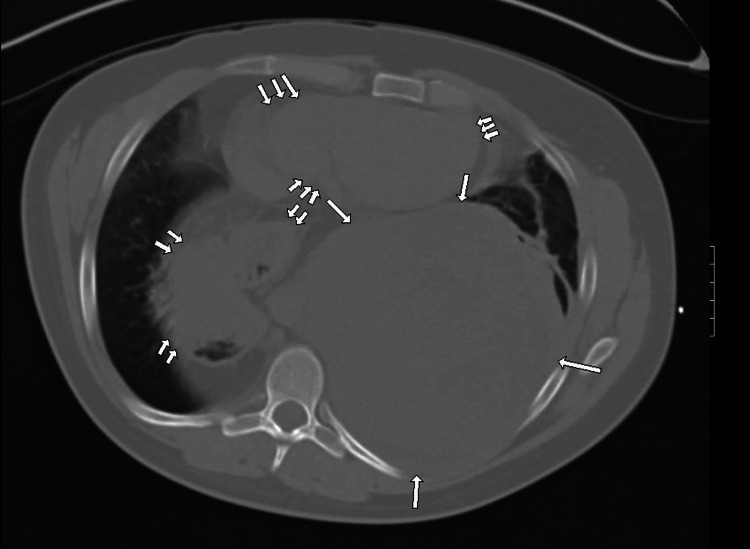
Non-enhanced chest CT axial image of the lower chest with lung window (with arrows) demonstrating the same findings noted in Figure 1 with a more detailed visualization of the air spaces
